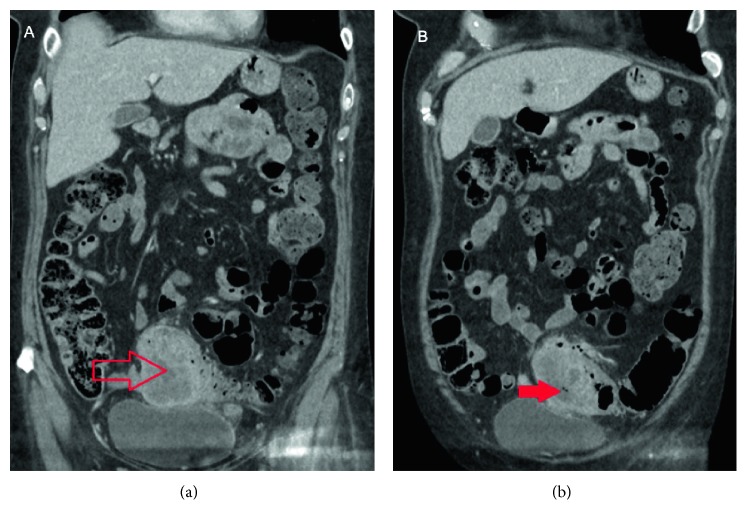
Coronal CT of the abdomen and pelvis highlighting an intramural sigmoid mass (a). Furthermore, the appearance of abscess transformation was noted, with gas locules evident (b).
